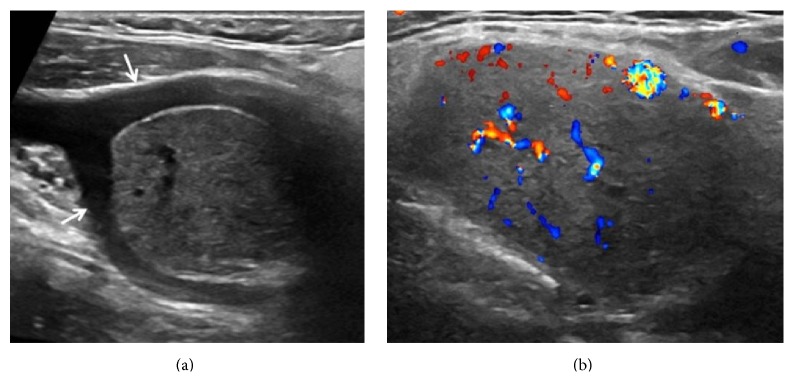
Gray-scale ultrasound (a) shows well defined, solid mass that causes splaying of the internal and external carotid arteries (arrows). High vascularity of lesions is seen in Doppler ultrasound image (b).
